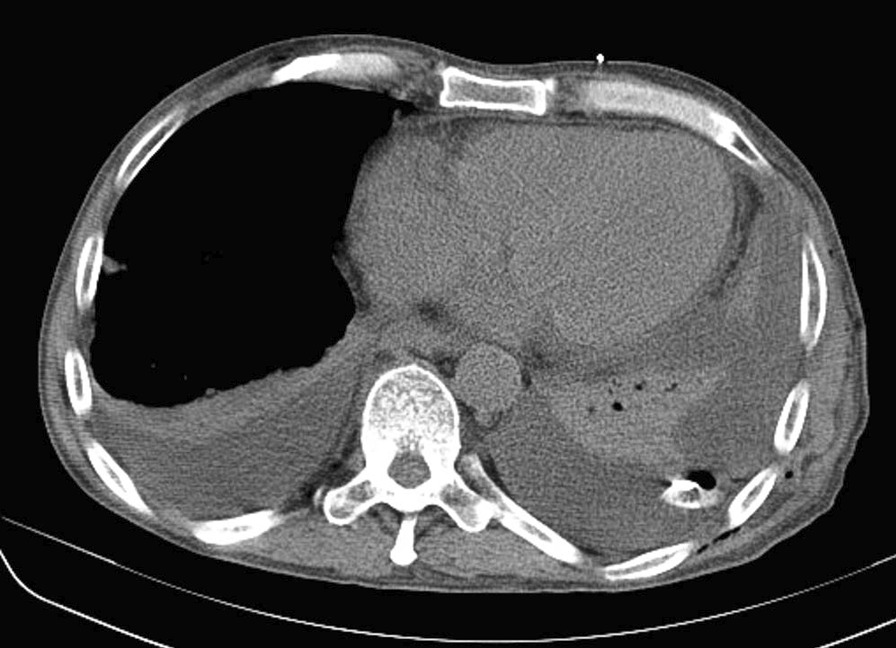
CT iamge of the thoracic drain insertion. A thoracic drain was placed in the chest cavity, but the pleural effusion was not drained.
